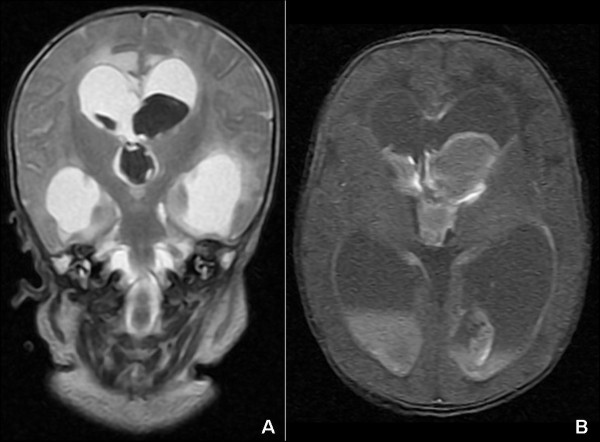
Neonatal intraventricular hemorrhage. MR images of subacute intraventricular hemorrhage in both lateral and third ventricles, causing intraventricular obstructive hydrocephalus. Layering fluid/fluid (fluid/heme) levels are present in occipital horns. Brainstem appears unremarkable. (Left: coronal T2WI, right: axial T1WI).
